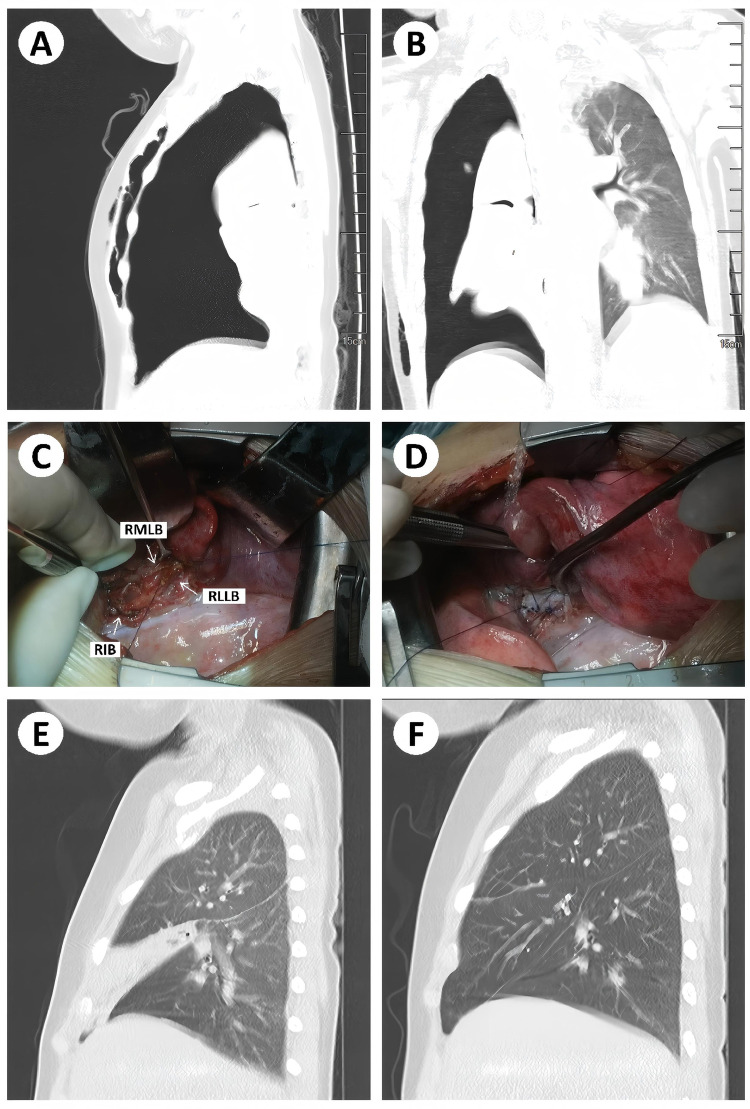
Traumatic case 5, a 3-year-old girl admitted following a traffic accident with coma. Surgery was performed after stabilization with closed thoracic drainage. (A,B) Preoperative CT images demonstrating right massive pneumothorax, pulmonary atelectasis, and an obscured right middle lobe bronchus. (C,D) Intraoperative findings revealed a fragmented transection of the distal bronchus intermedius, followed by mobilization and anastomosis of the right middle and lower lobe bronchi. Intraoperative bronchoscopy confirmed anastomotic patency. (E) CT at 2 weeks postoperatively showed atelectasis of the right middle lobe; bronchoscopy identified anastomotic granulation tissue, which was treated with local cryotherapy. (F) Three-month postoperative CT indicated restored bronchial lumen and satisfactory re-expansion of the middle lobe.
